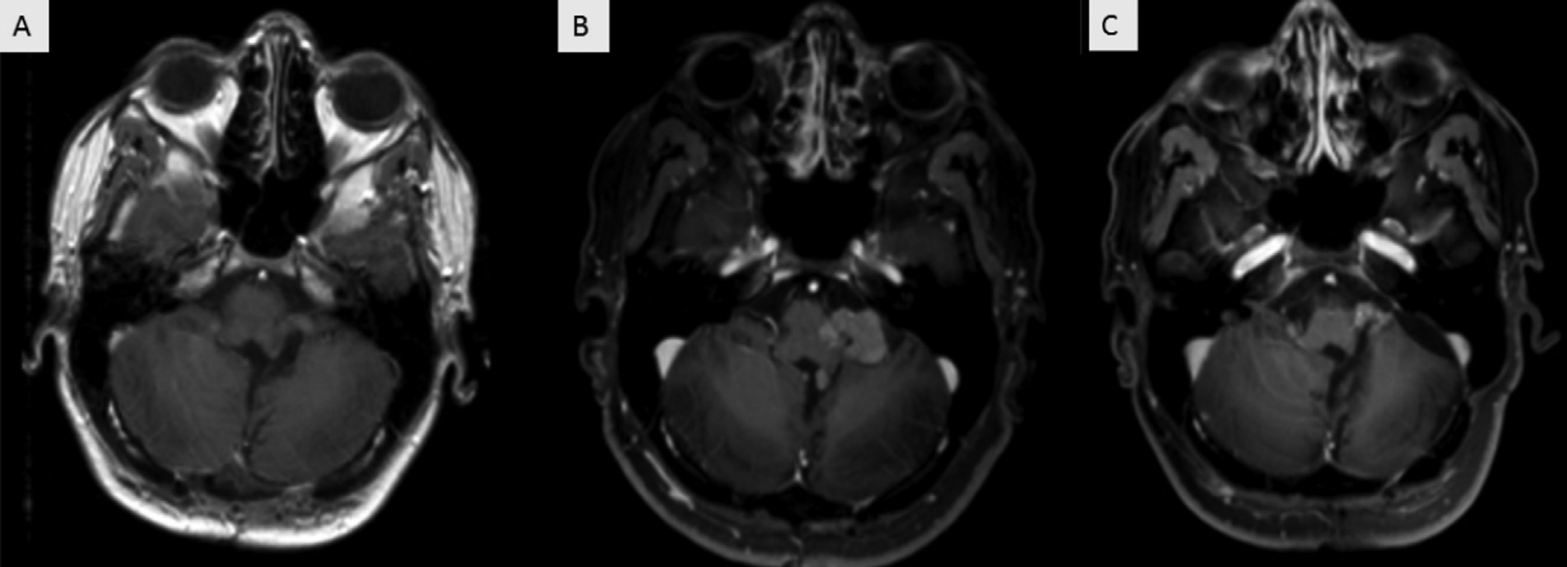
Postoperative T1 WI axial MRI scan one month (
